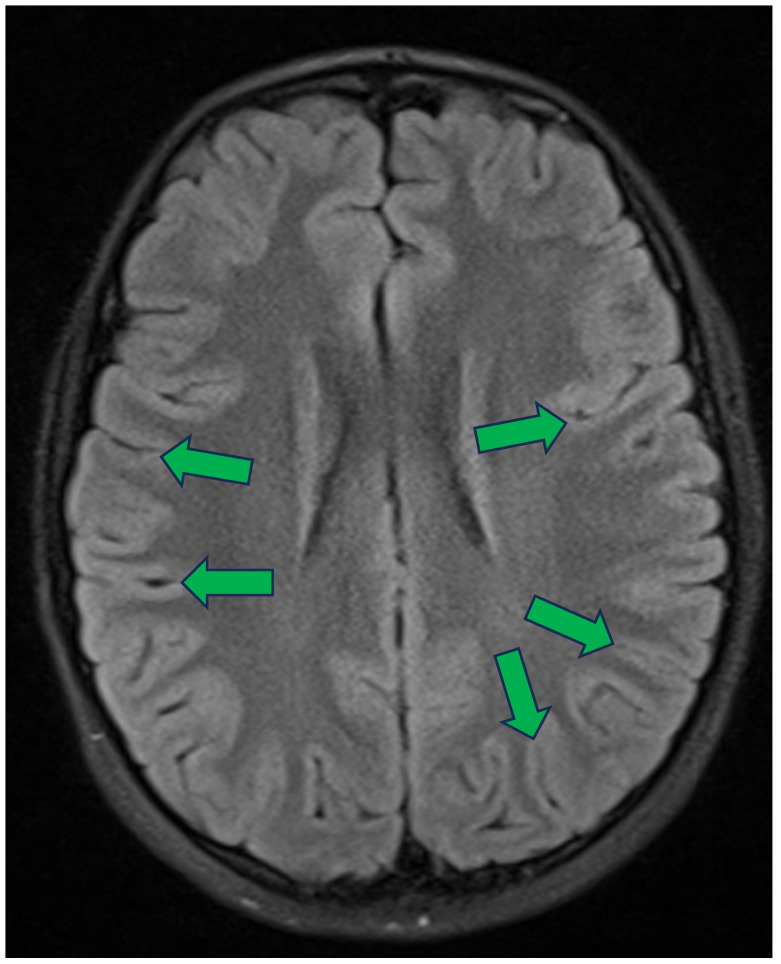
Axial section of fluid-attenuated inversion recovery (FLAIR) sequence of brain MRI of a young girl [28]. Numerous sulci are visible, with most appearing to form an angle close to a right angle relative to the skull and the scalp. Green arrows ending at the deepest visible point of several sulci oriented perpendicularly to the surface of the head.
